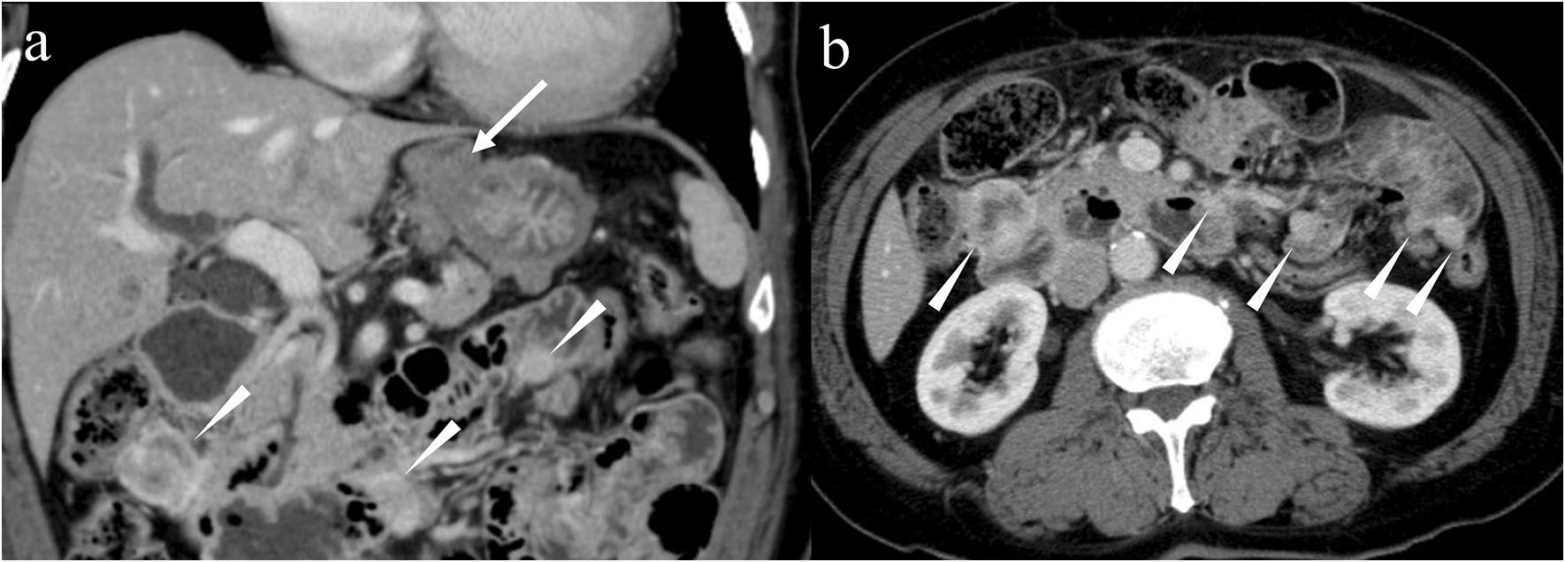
Multiple gastrointestinal stromal tumors associated with a familial gastrointestinal stromal tumor. A 78-year-old male presented with anemia. Coronal and axial contrast-enhanced CT in the venous phase demonstrate a homogeneous hypovascular tumor in the stomach (a: arrow) and multiple homogeneous or heterogeneous hypervascular tumors in the duodenum and jejunum (a and b: arrowheads). Diverse imaging findings (i.e., hypovascular vs. hypervascular and homogeneous vs. heterogeneous) are observed even in the same patient
